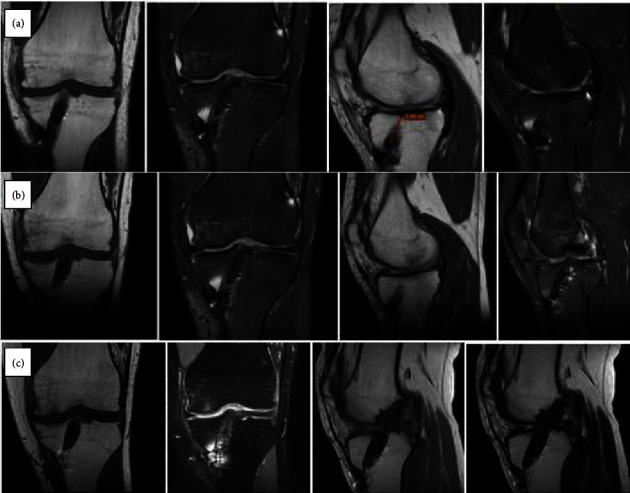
MRI graft images of a case during the 4th (a), 8th (b), and 12th (c) month follow-up.
